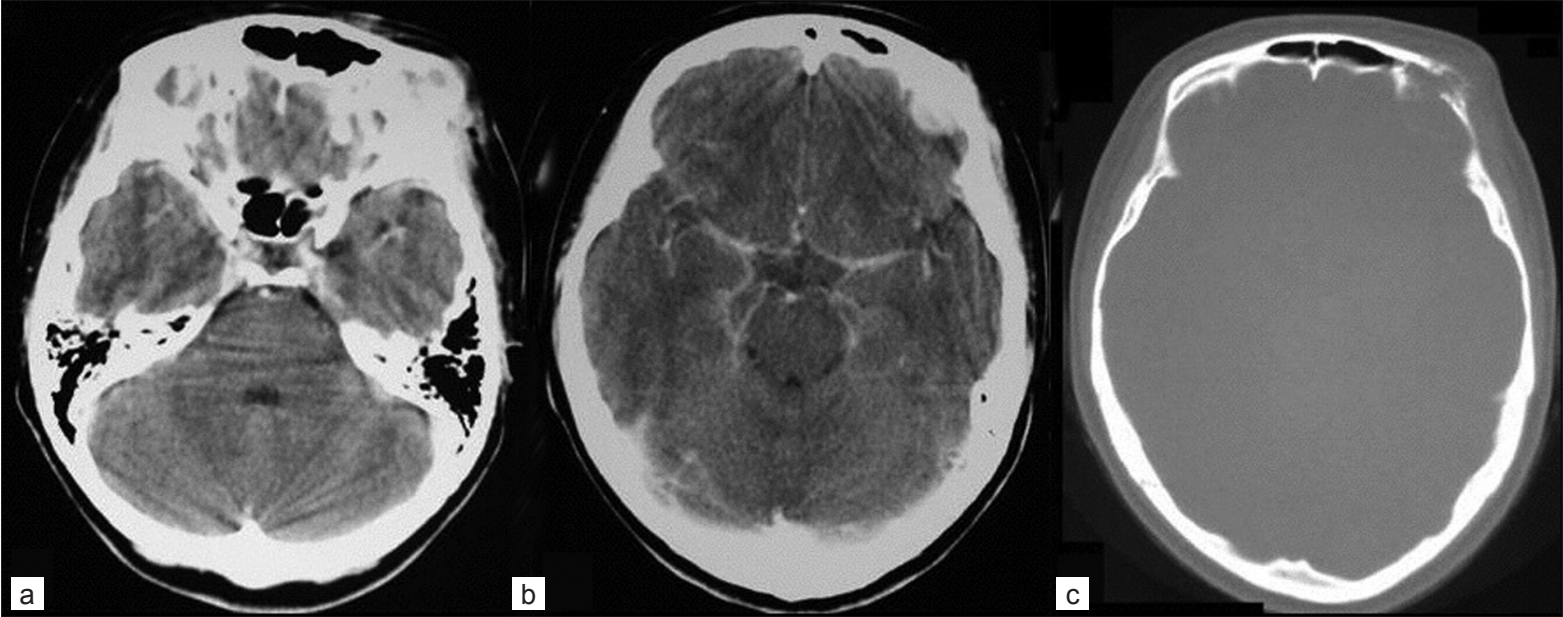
MRI study showing a) an isointense 3.5 × 2.5 cm mass on axial T1-weighted image, b) T1 with contrast, c) hyperintense mass with marked border to its surrounding and appearance of bone destruction on T2 weighted image
